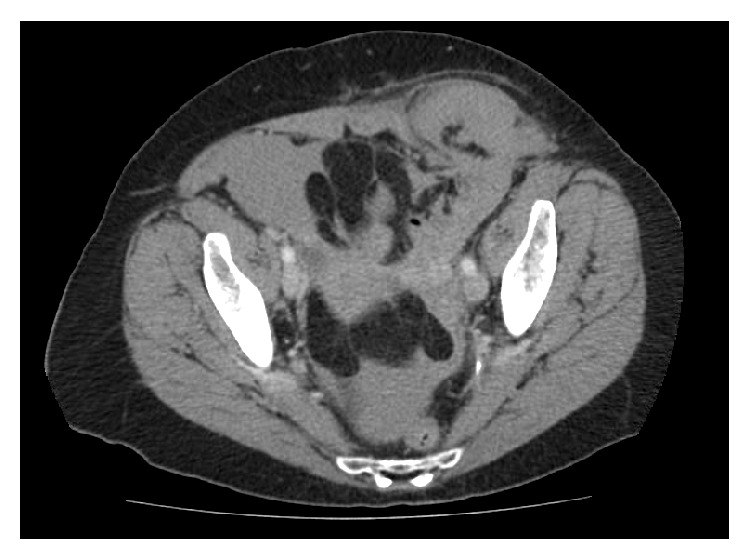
Preoperative noncontrast abdominal CT scan showing a large left indirect inguinal hernia sac.
